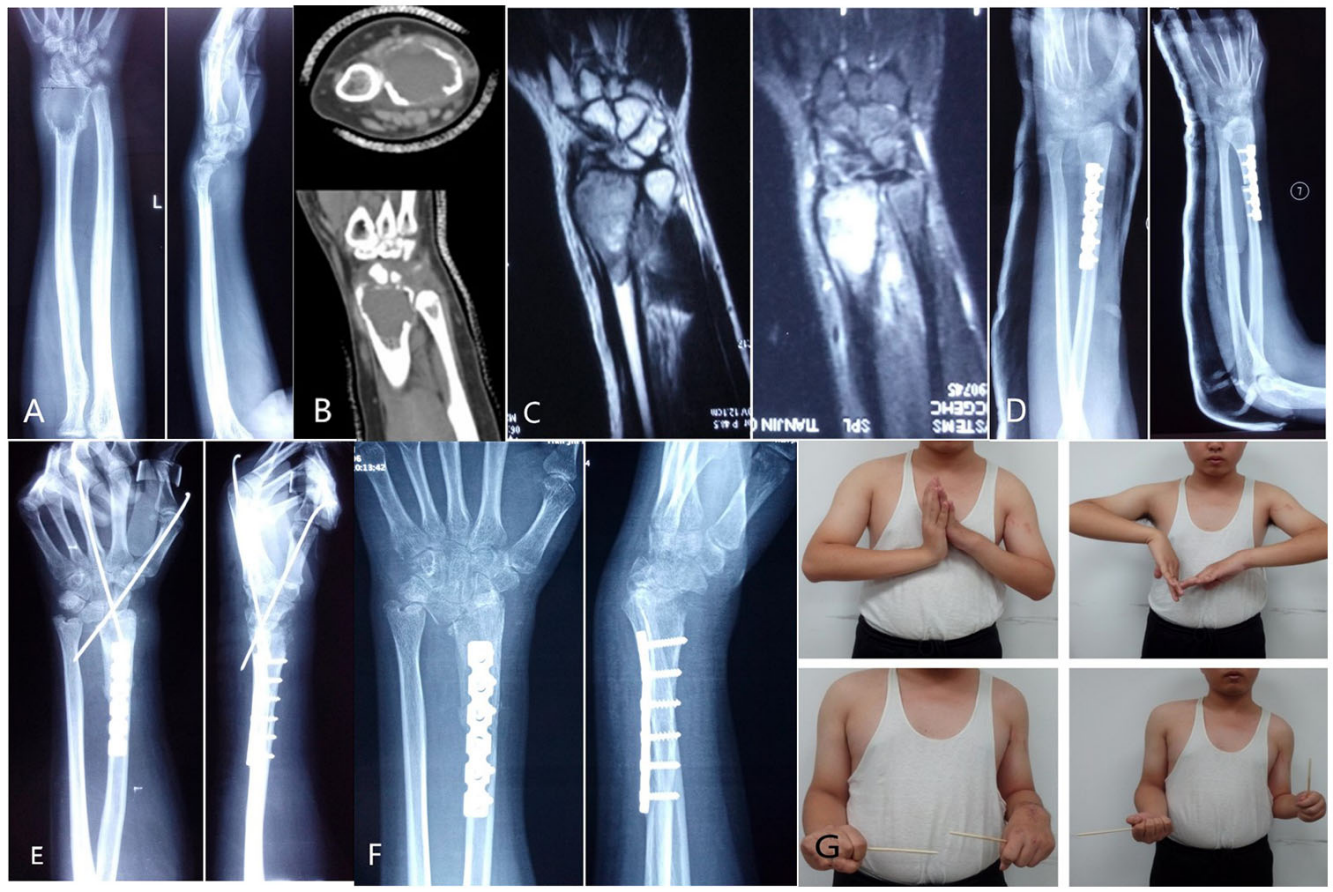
(A) The first preoperative X-ray film showed expansive bone destruction at the distal end of the left radius, discontinuous bone cortex, and volar subluxation of the radiocarpal joint. (B) The first preoperative CT showed that the metacarpal and dorsal bone cortex of the distal radius were perforated and the articular surface was involved. (C) The first preoperative MRI showed that the tumor invaded the ulnar soft tissue with unclear boundary. (D) After resection of tumor segment and reconstruction of wrist joint with autologous fibular head transplantation, X-ray showed that the fracture line was clear and the broken end was well aligned, and the wrist joint was fixed with external fixation brace. (E) Local recurrence and dorsal dislocation of wrist joint 2 years after operation. Secondary tumor resection, partial resection of extensor pollicis longus tendon, transposition and repair of extensor digitorum tendon, wrist reduction and Kirschner wire fixation. (F) The X-ray image 39 months after surgery showed radiocarpal arthritis (grade 3), distal radioulnar joint separation, wrist ulnar deviation and dorsal dislocation. (G) The patient was followed up for 39 months and showed poor wrist flexion, extension and supination.
